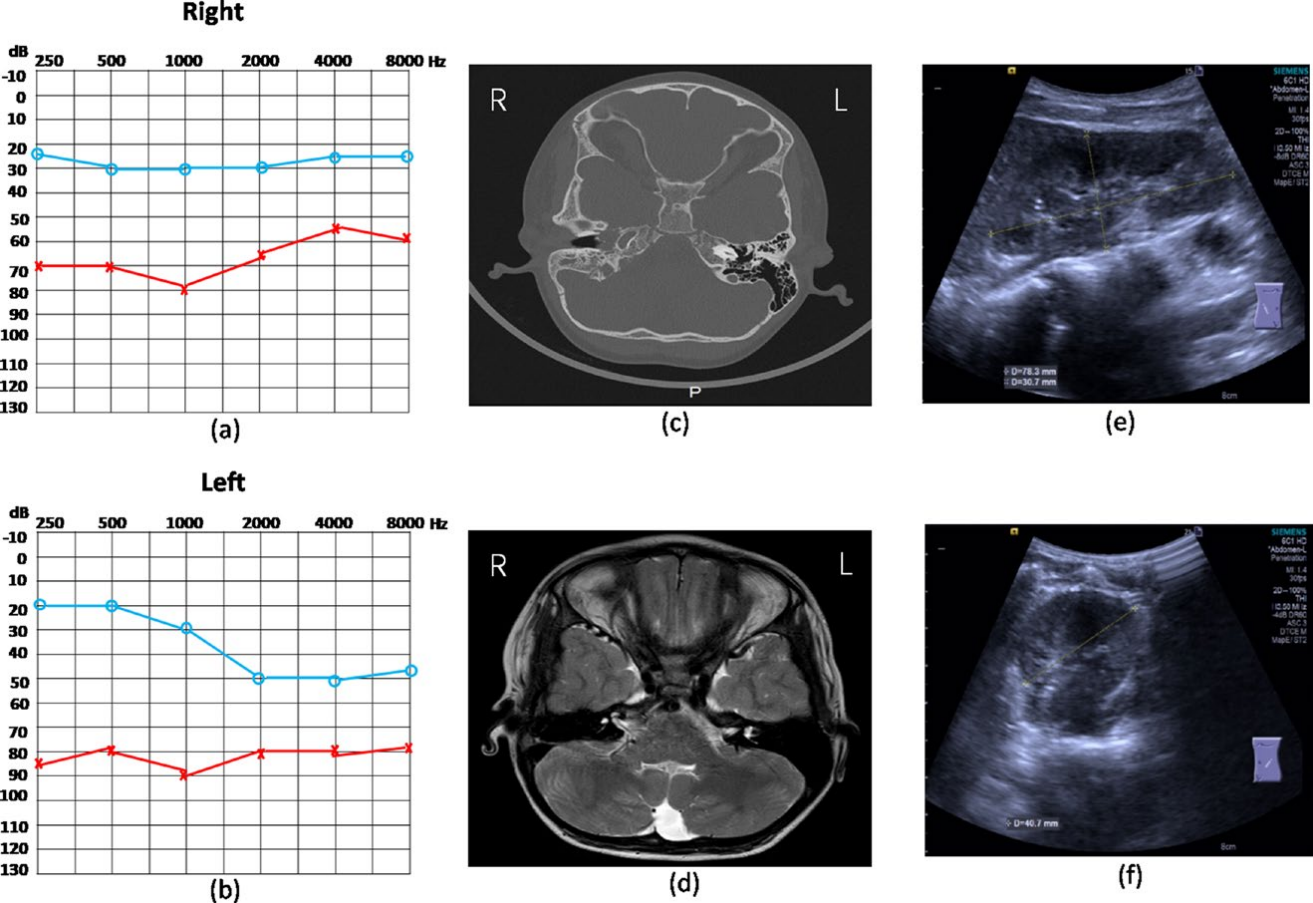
The phenotypes of the proband (III: 4, female, 7 years old) (a, b) Pure tone bone and air conduction thresholds of the right ear (a) and left ear (b) were presented, respectively. Abscissa represents frequency (Hz); Ordinates represent decibel (db). (c) CT scan data showed the ossicular chain malformation in the left ear. (d) Based on the MRI data, the cochlea was well developed. Additionally, both the internal auditory meatus and the membranous labyrinth were well developed. (e, f) Renal ultrasound data showed the right kidney (e) and the left kidney (f) were well developed
